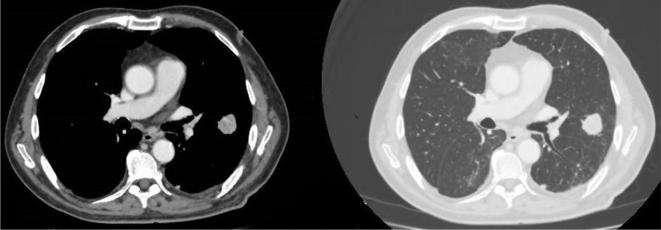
Thoracic CT. Pulmonary, solid, and bilateral nodules with no pleural effusion.
